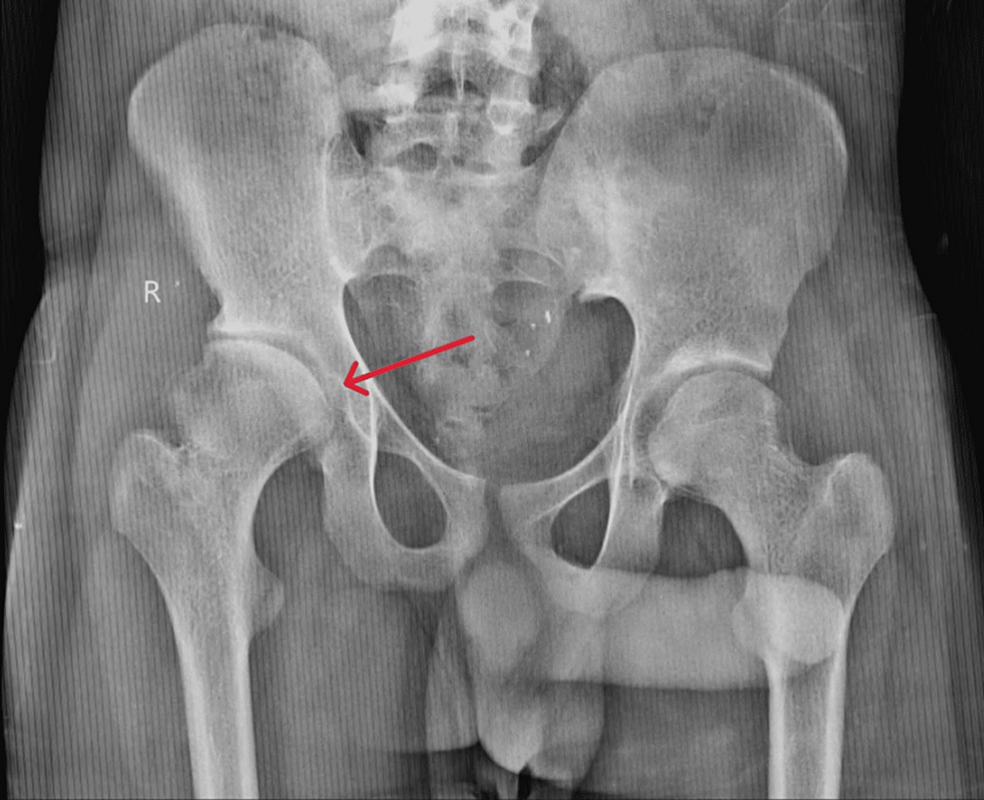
An X-ray of the pelvis and both hips showing bony irregularities in the contour of the right hip joint (red arrow)
